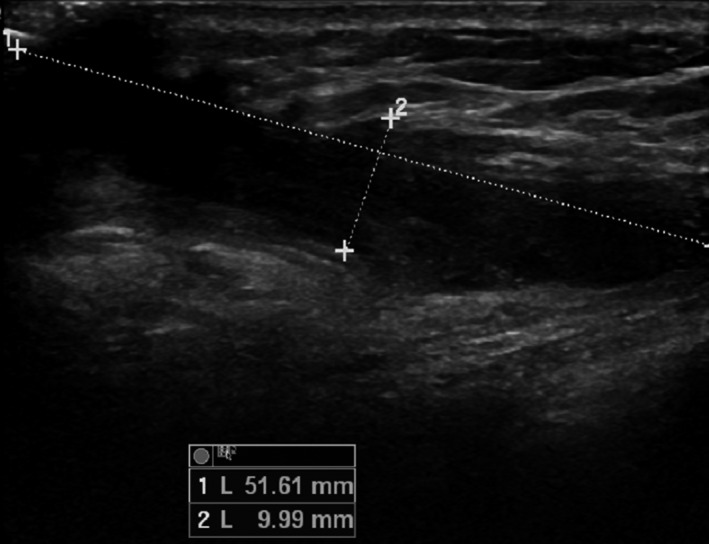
Ultrasound, sagittal cut, showing the 5 × 10 cm length of the fistulous track.
